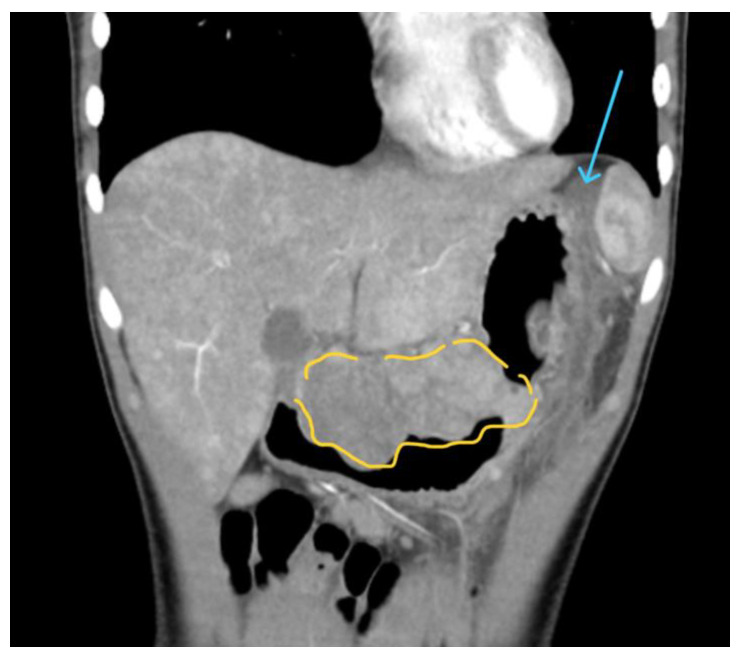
Gastric tumor-GIST seen in CT scan before biopsy was obtained. In yellow you can see a large tumor mass. The blue arrow points to ascites present in the abdomen.
